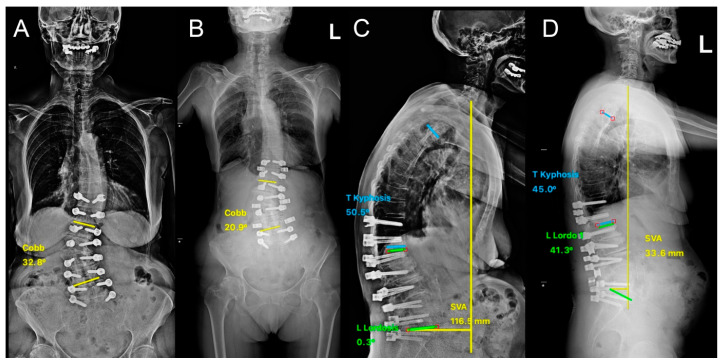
Patient with spinal stenosis and mixed coronal-sagittal deformity presented to the clinic with lower back pain and neurogenic claudication. The patient was previously diagnosed with osteoporosis (T: −2.9). Two-stage dynamic stabilization with Safinaz and PEEK rods was performed. Standing anteroposterior X-rays (A) before and (B) after the second-stage surgery showing improvement in the coronal deformity. Standing lateral X-rays (C) before and (D) after the second-stage surgery demonstrate significant improvement in the sagittal alignment.
